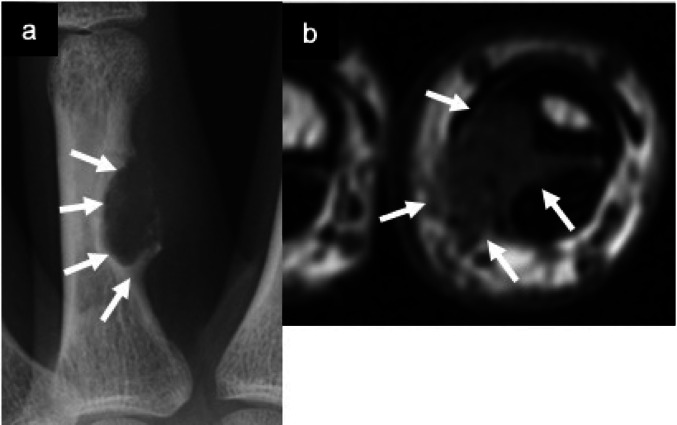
Localized type tenosynovial giant cell tumor. Plain radiograph of the finger (a) shows a soft tissue mass associated with pressure induced bony erosion and a surrounding sclerotic rim at the proximal phalanx (white arrows). Axial fat-suppressed T1-weighted image (b) demonstrates a soft tissue mass with corresponding bony erosion (white arrows)
